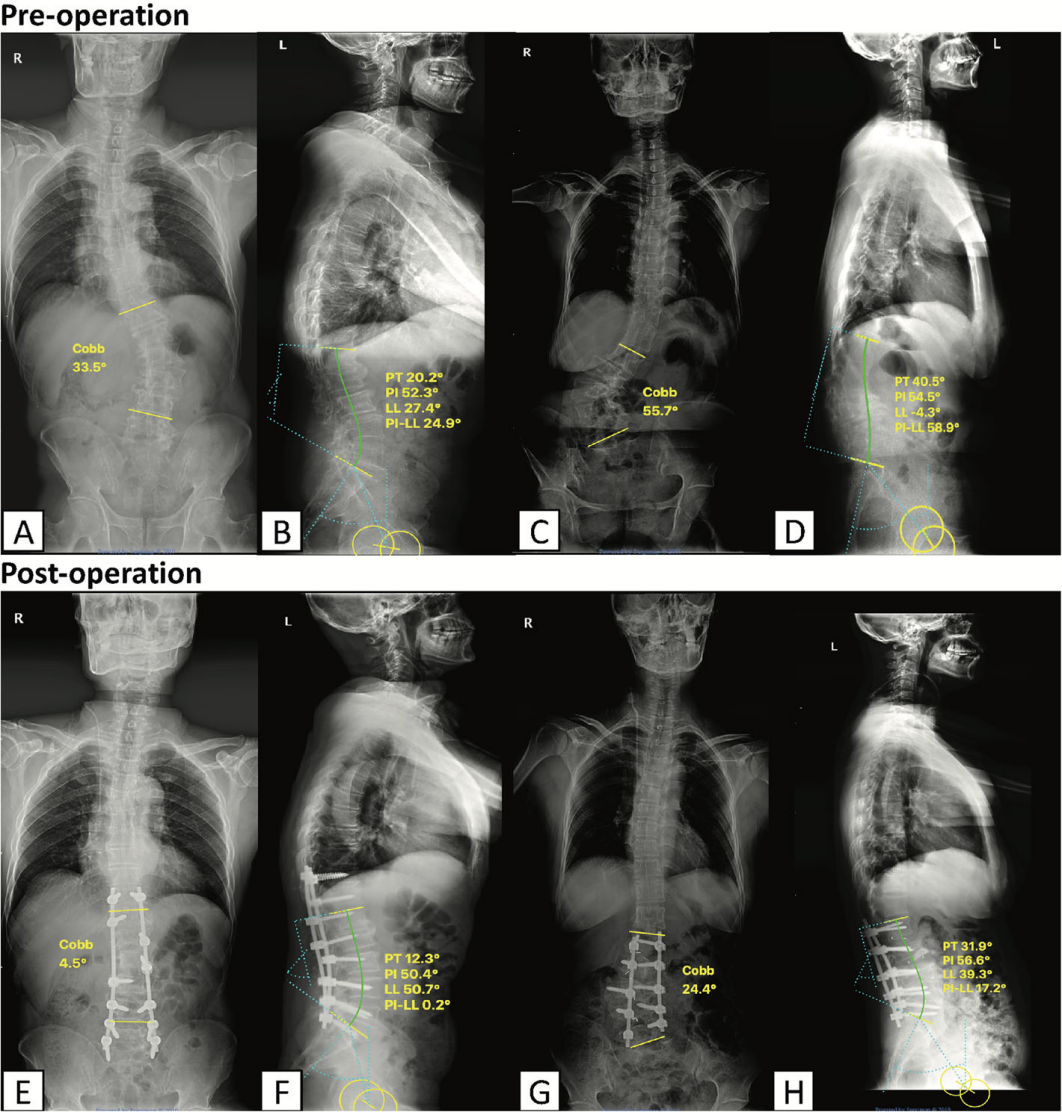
Pre-operative (a–d) and post-operative (e–h) radiographs of two cases including a 61-year-old male (a, b, and e, f) and a 48-year-old female (c, d and g, h) with degenerative scoliosis were shown in this figure. Both of them underwent robot-assisted scoliosis correction surgery. The average operative time was 240 min, and mean blood loss was 400 ml. Symptoms including unstable gait and lower limbs numbness improved after surgeries
